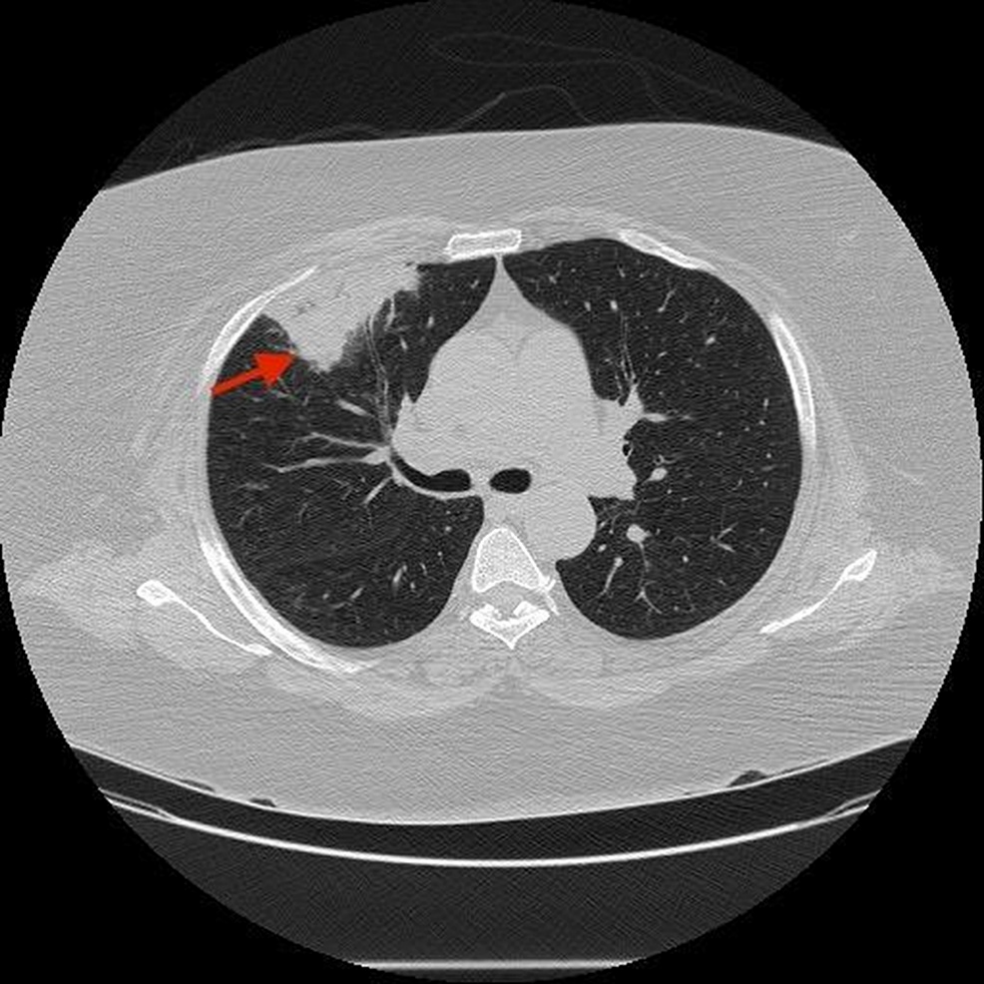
CT chest showed well-demarcated area of consolidation in the peripheral right upper lobe which could represent an area of infarction
